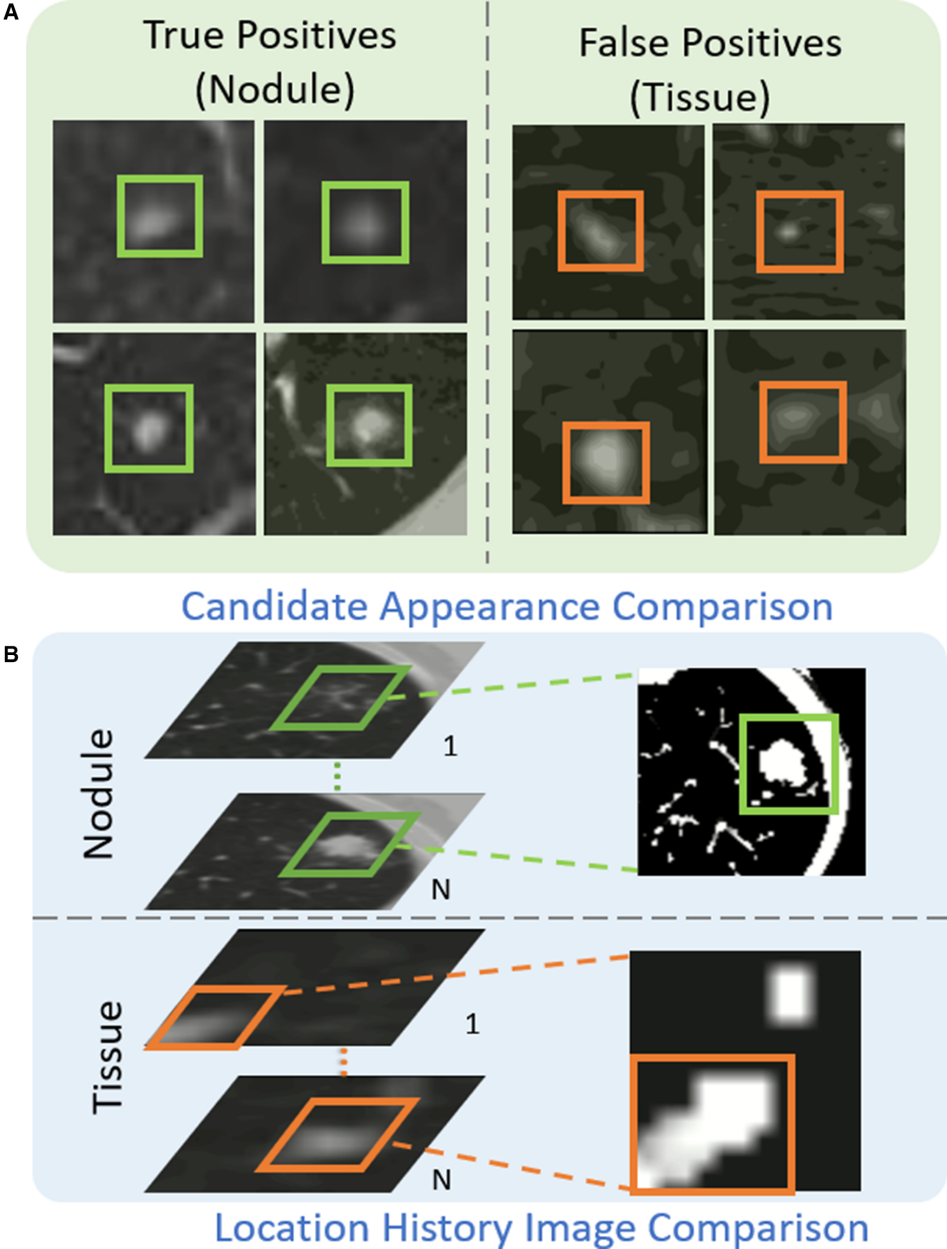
The proposed Location History Images (LHI) to distinguish tissues and nodules from the predicted nodule candidates. (A) The true nodules (green boxes) have similar appearances to the false detected tissues (orange boxes). (B) The location variances for nodules and tissues are oriented differently in LHIs. True nodules generally have a circular region representing the spatial changes as the brighter center (the size of the nodules decreases in the following CT slides) or a darker center (the size of the nodules increases in the following CT slides). On the other hand, the location variance for false detected tissues tends to change in certain directions, such as a gradual change of the trajectory line.
